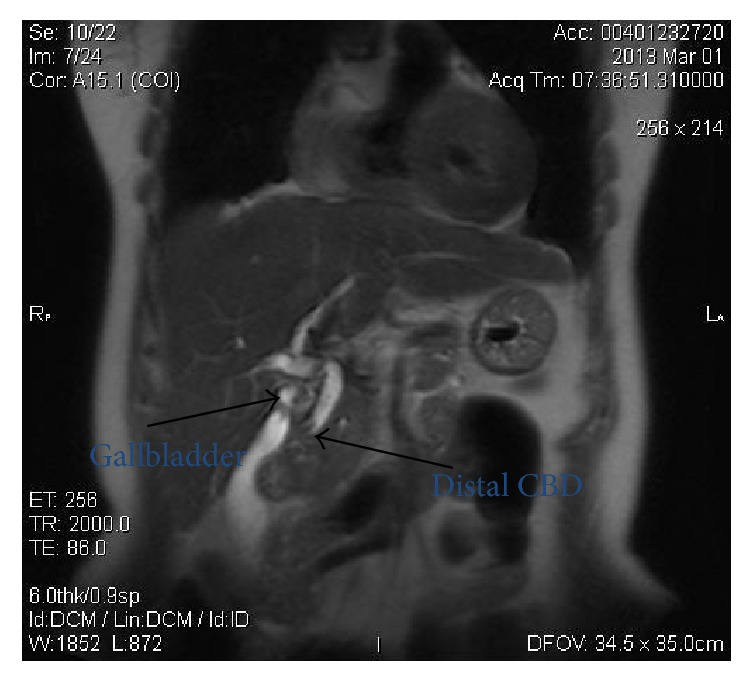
MRI: coronal section shows gallstones and the pointed filling failure in the distal common bile duct.
